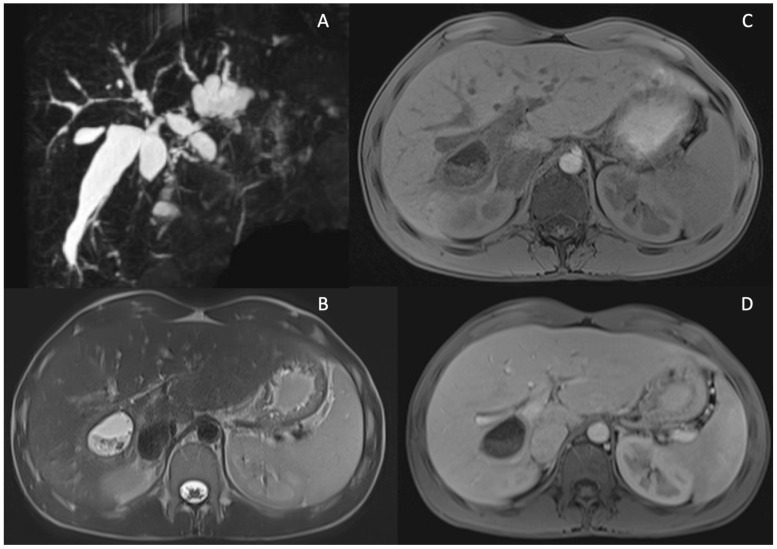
Cystic dilatations (CD) of intrahepatic bile ducts in PSC patients. MRCP sequence showing diffuse cystic dilatations of intrahepatic bile duct (A). MRI showing a large CD of intrahepatic bile duct dilatation localized between S5 and S6 filled with stones in T2 w images (B), T1w fat sat pre-contrast (C) and after Gd-EOB-DTPA (portal phase) showing wall thickening and enhancement of the CD (D).
